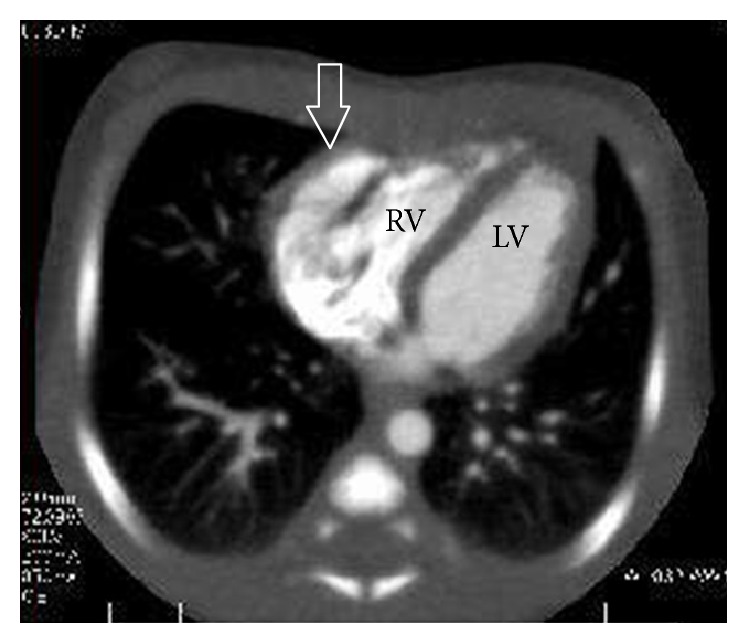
Postnatal computed tomography scan showing myocardium in the diverticulum arising from the right ventricle. LV, left ventricle; RV, right ventricle. Arrow indicates the diverticulum.
